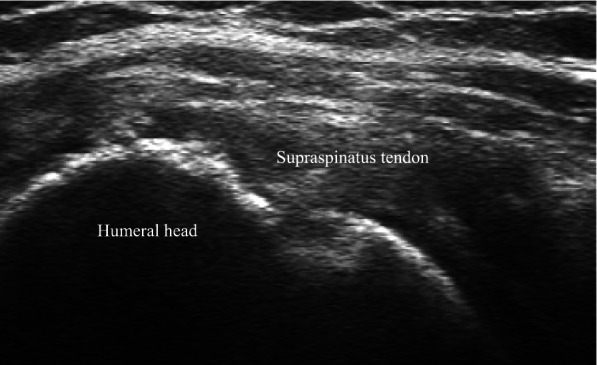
Post-operative six-month follow-up ultrasonography: the integrity of the repaired rotator cuff tendon was intact
